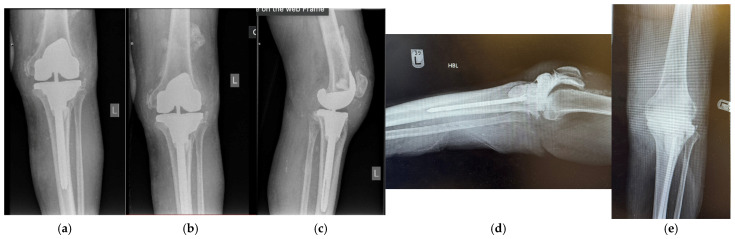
Post-operative X-rays for case 5: (a–c) spacer following first-stage revision and (d,e) radiographic findings upon completion.
